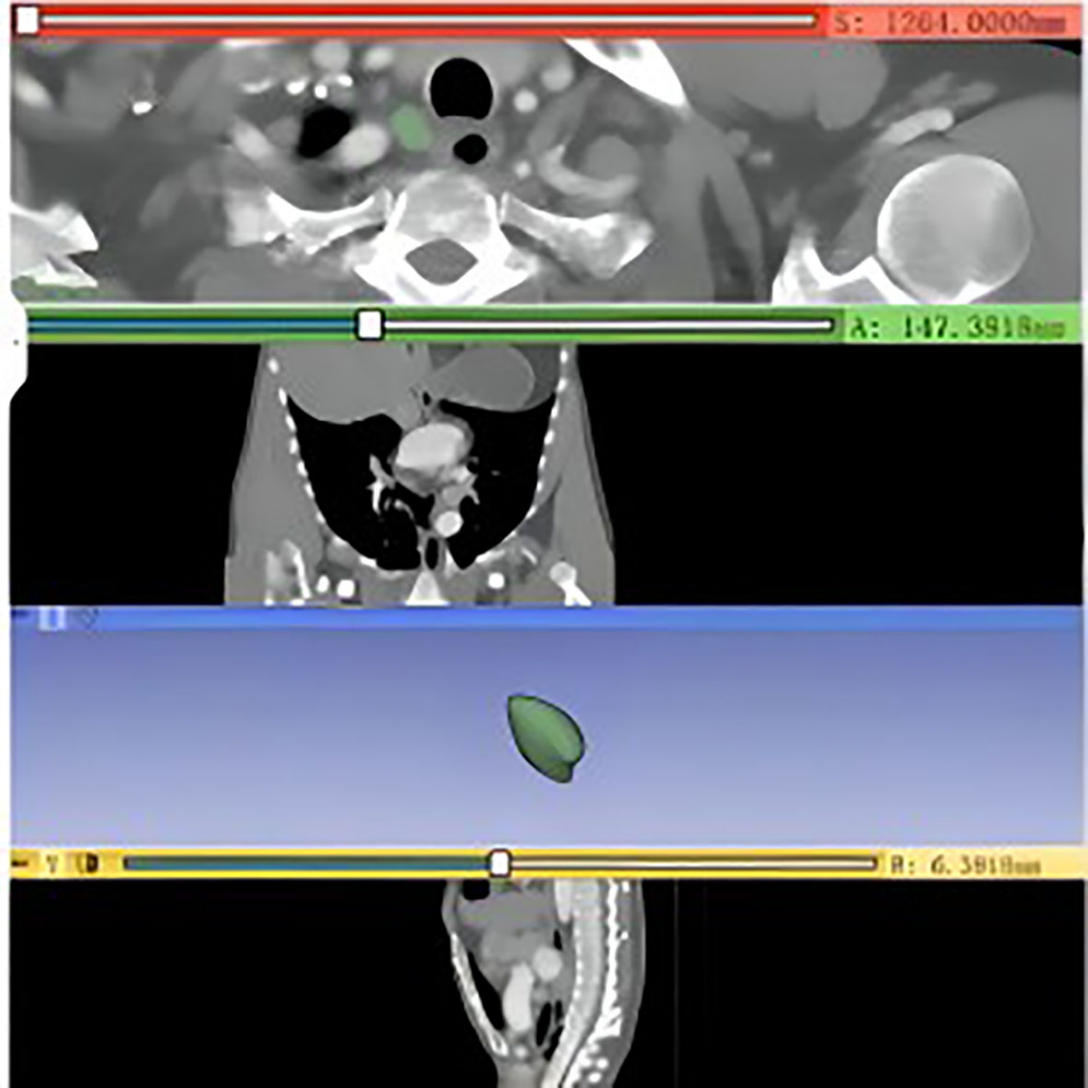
CT 3D reconstruction of the RRLNLN.
